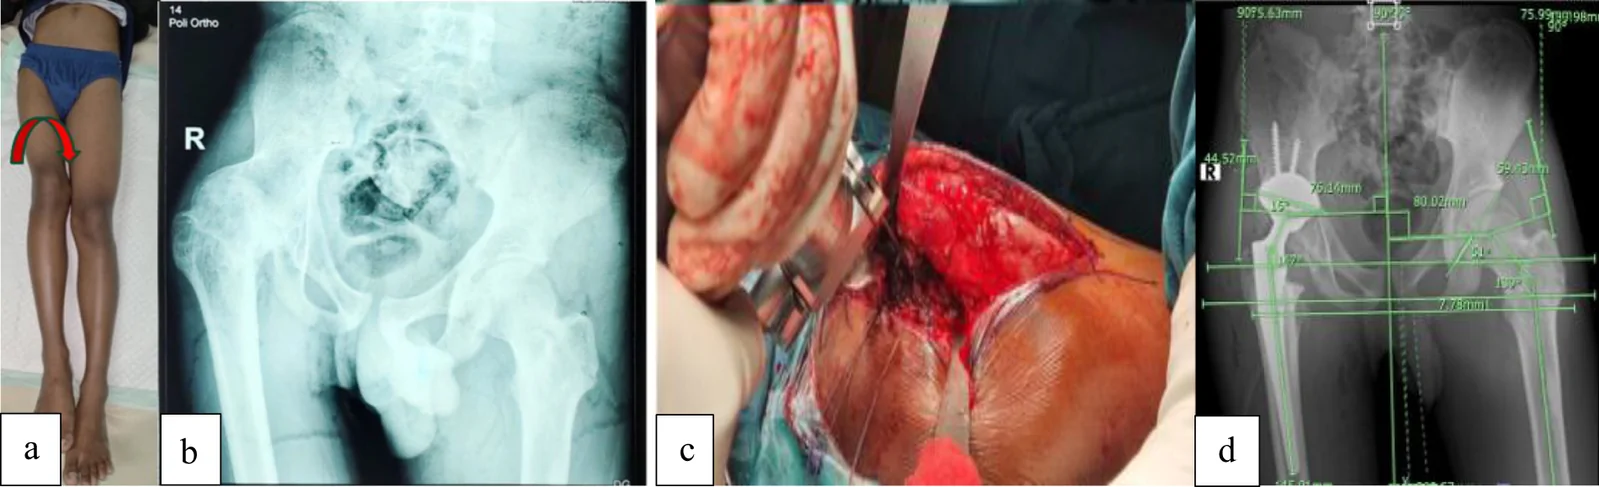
Preoperative, intraoperative, and postoperative clinical and radiographic findings for Case 2. (a) Clinical appearance before surgery, showing right lower-limb deformity, shortening, and internal rotation of the hip. (b) Pelvic radiograph before surgery, showing right hip ankylosis. (c) Intraoperative view during THA through the posterior approach. (d) Pelvic radiograph after surgery.
